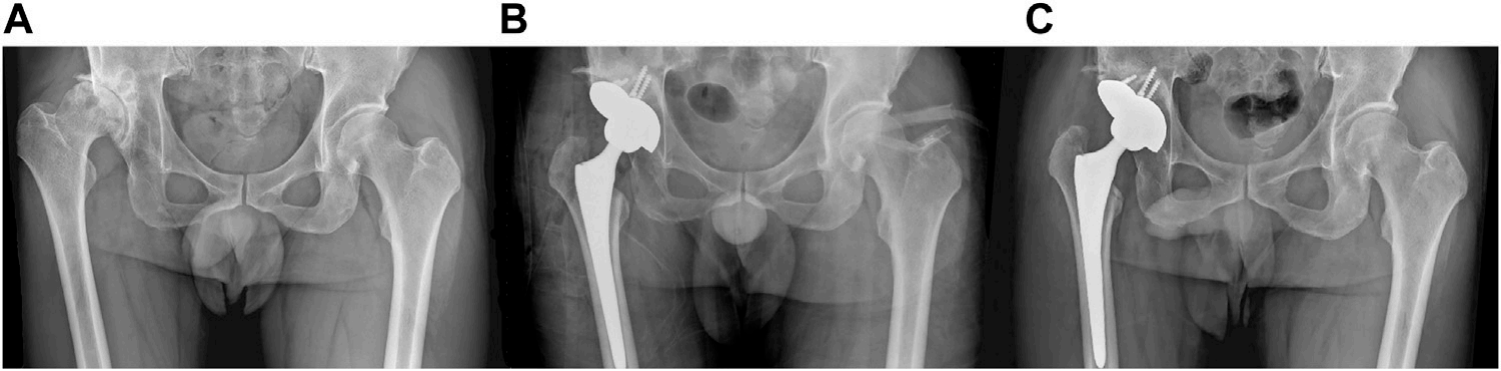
A 65-year-old male patient with Crowe III DDH who underwent 3D-printed IAP acetabular reconstruction. (A) Preoperative X-ray image; (B) immediate postoperative X-ray image; (C) postoperative last follow-up X-ray image.
